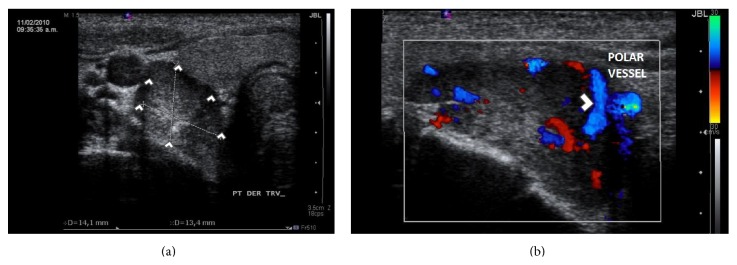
Neck ultrasonography on admission. (a) Large echogenic mass dorsal to the right lobe of the thyroid gland. (b) Doppler effect showing polar vessel finding, present in most adenomas. Findings are marked with white arrow heads.
